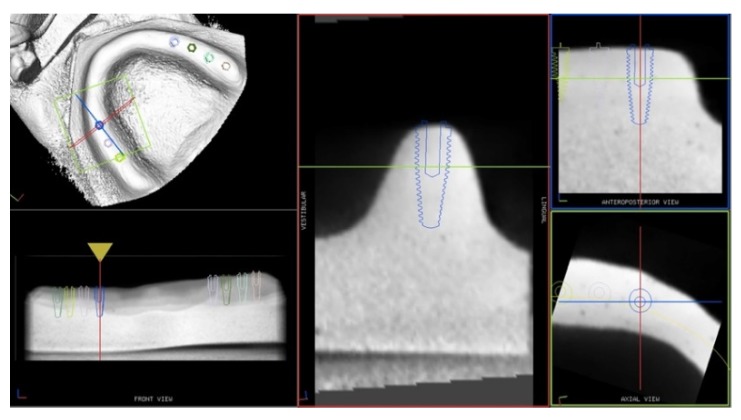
Plan of the implants position on the model CBCT images.
